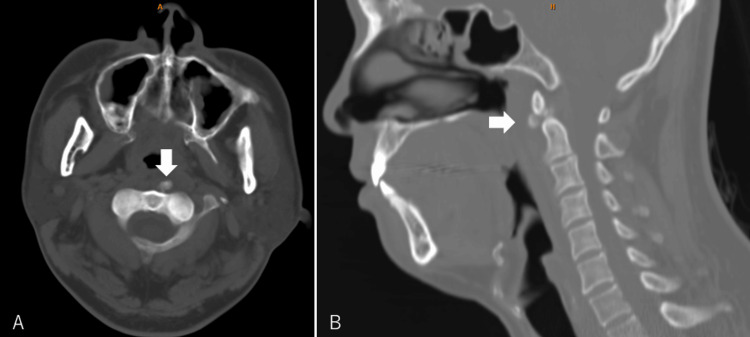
(A) A horizontal view of the neck CT. An arrow indicates the calcification of the longus colli muscle. (B) A sagittal view. An arrow indicates the calcification CT, computed tomography
